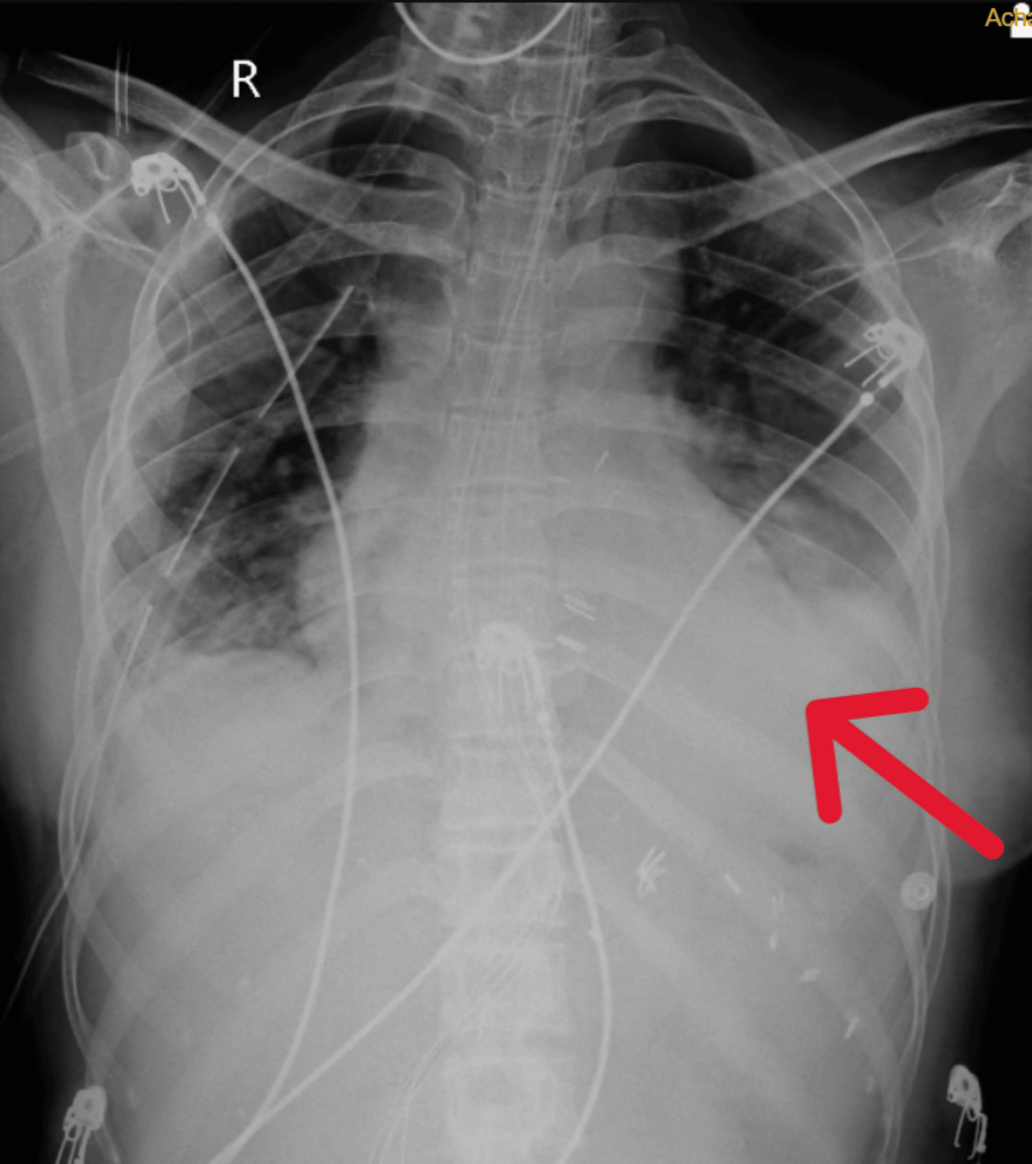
X-ray PA view of pre-rehabilitation The red arrow shows homogenous opacity. PA: posteroanterior view
